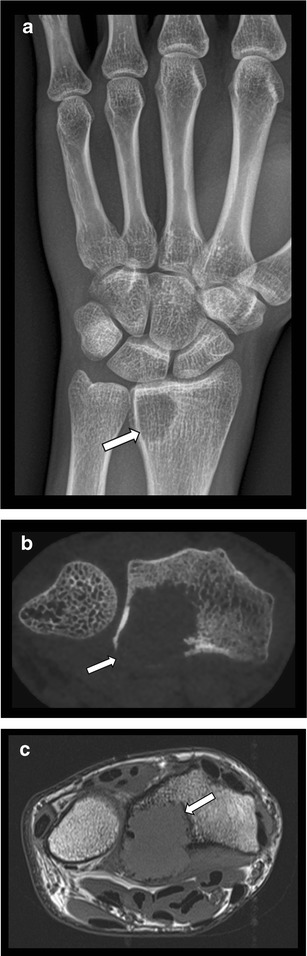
Giant cell tumour of distal radius. a. CR (AP view) showing an osteolytic lesion in the distal epiphysis of the radius (arrow). There is no major cortical breakthrough visible on CR. b. Axial CBCT reconstruction clearly show cortical breakthrough of the lesion. The precise extent of the soft tissue involvement is inaccurate due to insufficient soft tissue contrast. c. On axial T1-WI MRI, the lesion is isointense to muscle with cortical disruption at the volar aspect and involvement of pronator quadratus muscle. MRI is far superior for evaluation of the soft tissue component of the lesion
